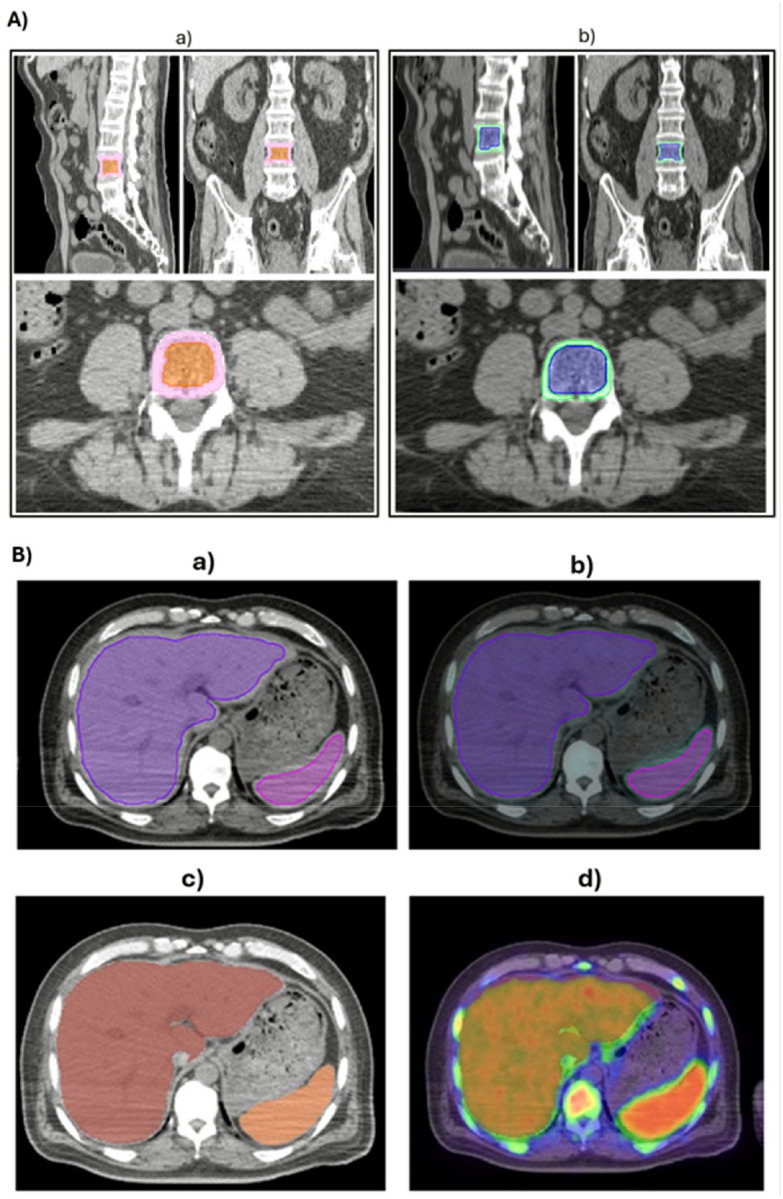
Overall segmentation of vertebral structures and Thoracoabdominal organs. A) Vertebral cortical and trabecular bone segmentation shown in axial, sagittal, and coronal views, including (a) automated segmentation and (b) manual segmentation. B)Liver and spleen segmentation on CT images, including (a) manual segmentation, (b) PET-CT map in the Velocity workspace, (c) automated segmentation, and (d) PET-CT map in the DAFS software.
